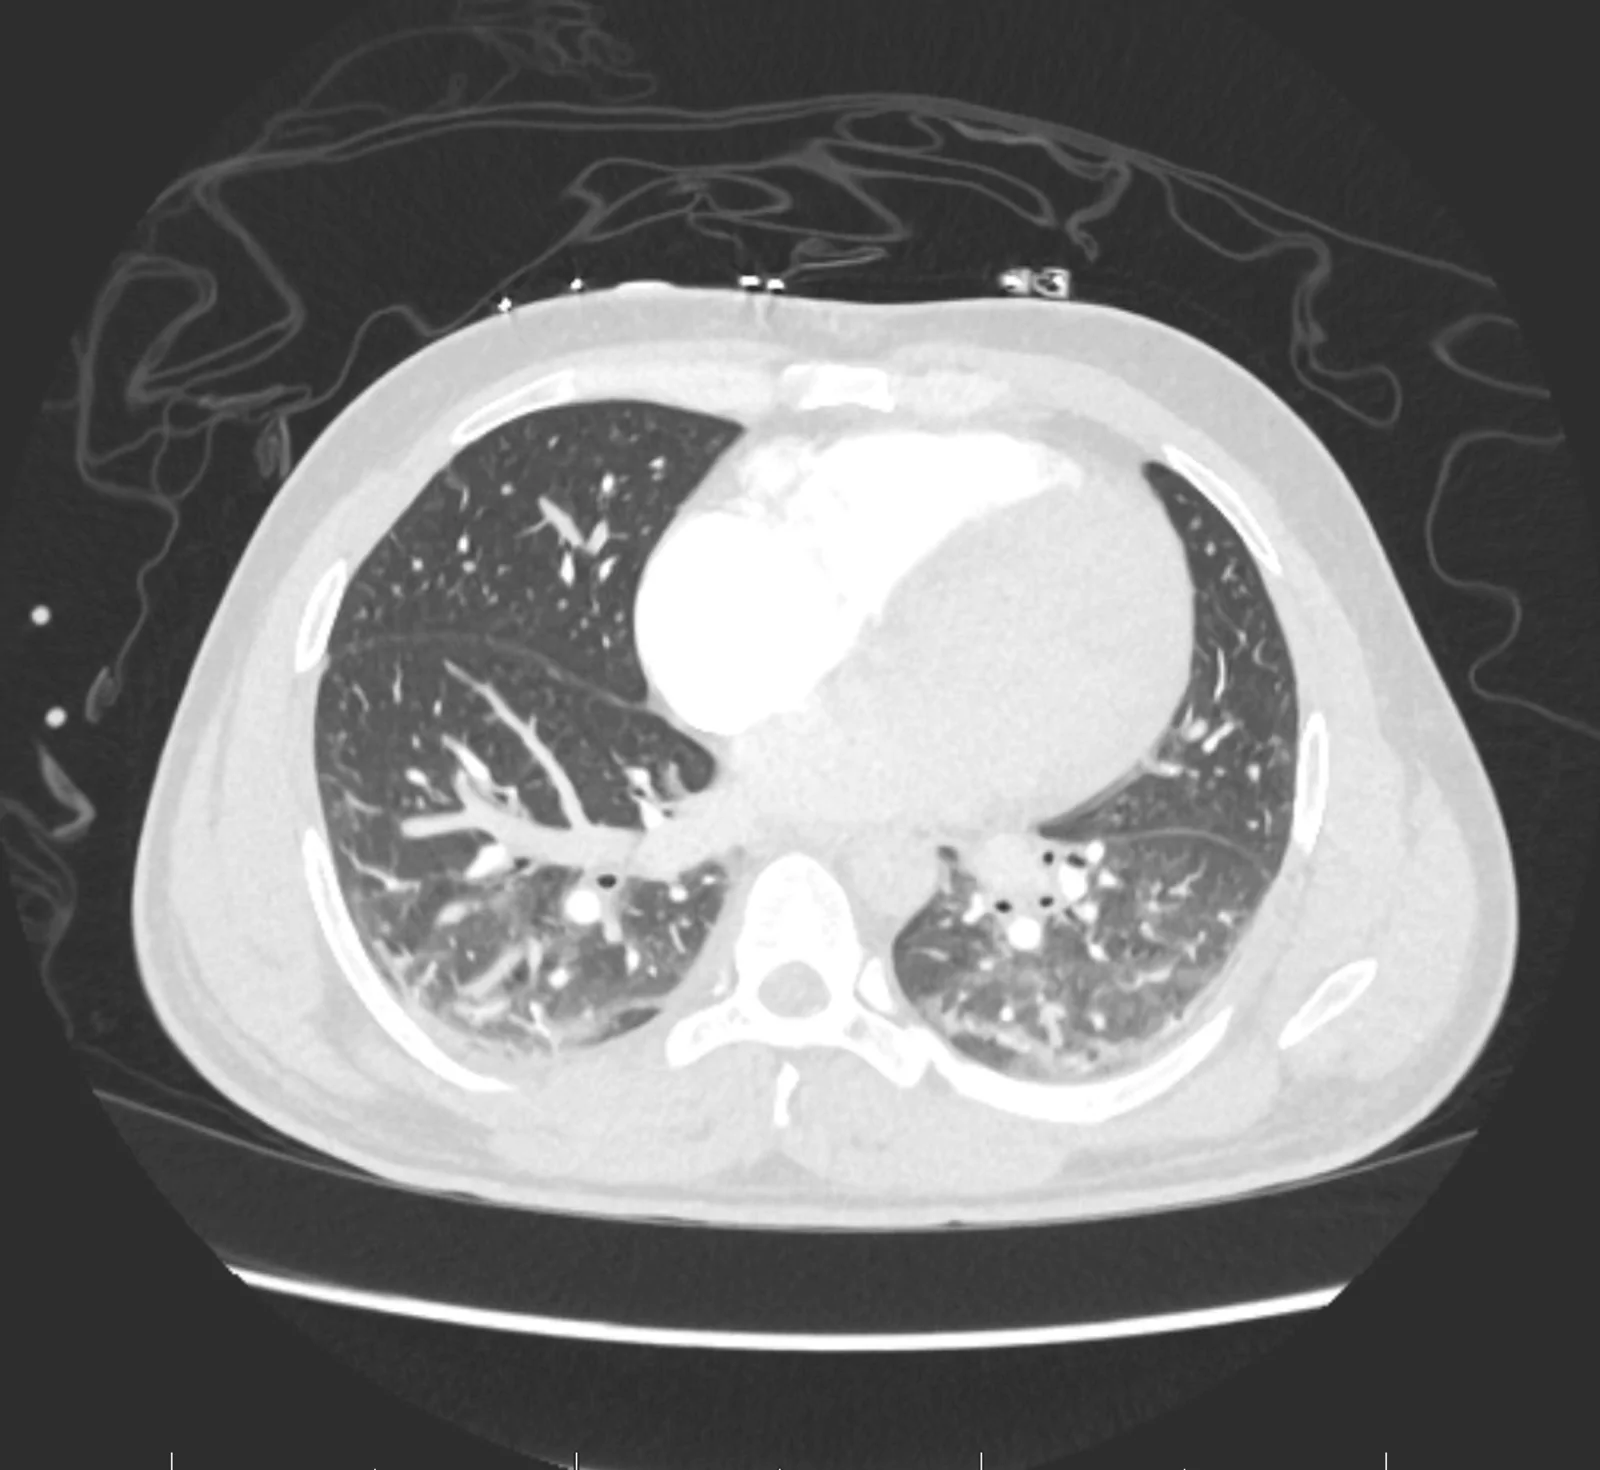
Chest computed tomography obtained after onset of hypoxic respiratory failure, demonstrating bilateral interstitial pulmonary edema and volume overload.
